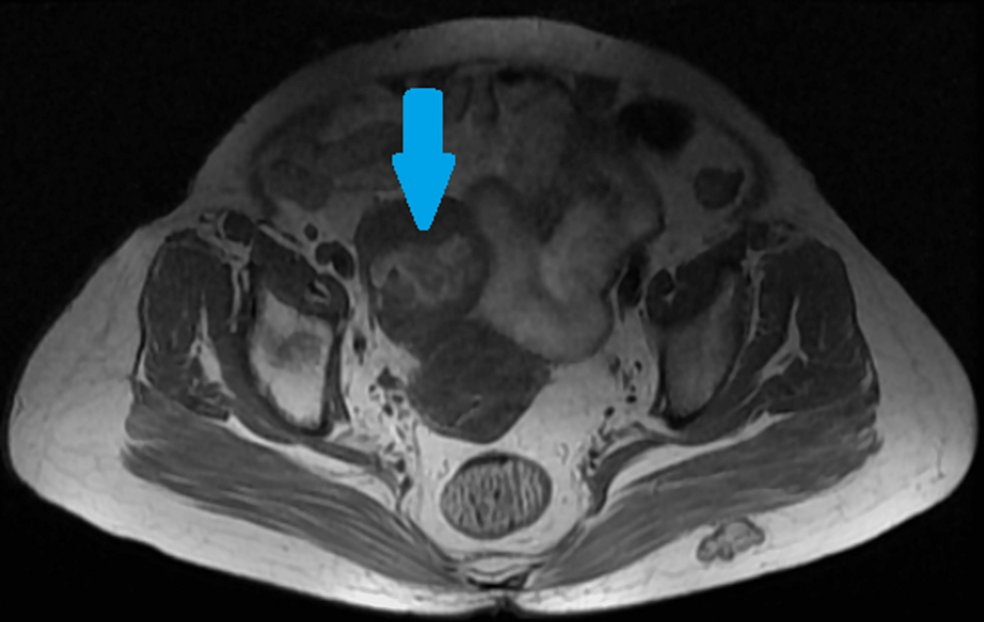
Magnetic resonance image showing an axial cut of the uterus with neoplastic ovarian mass (blue arrow)
